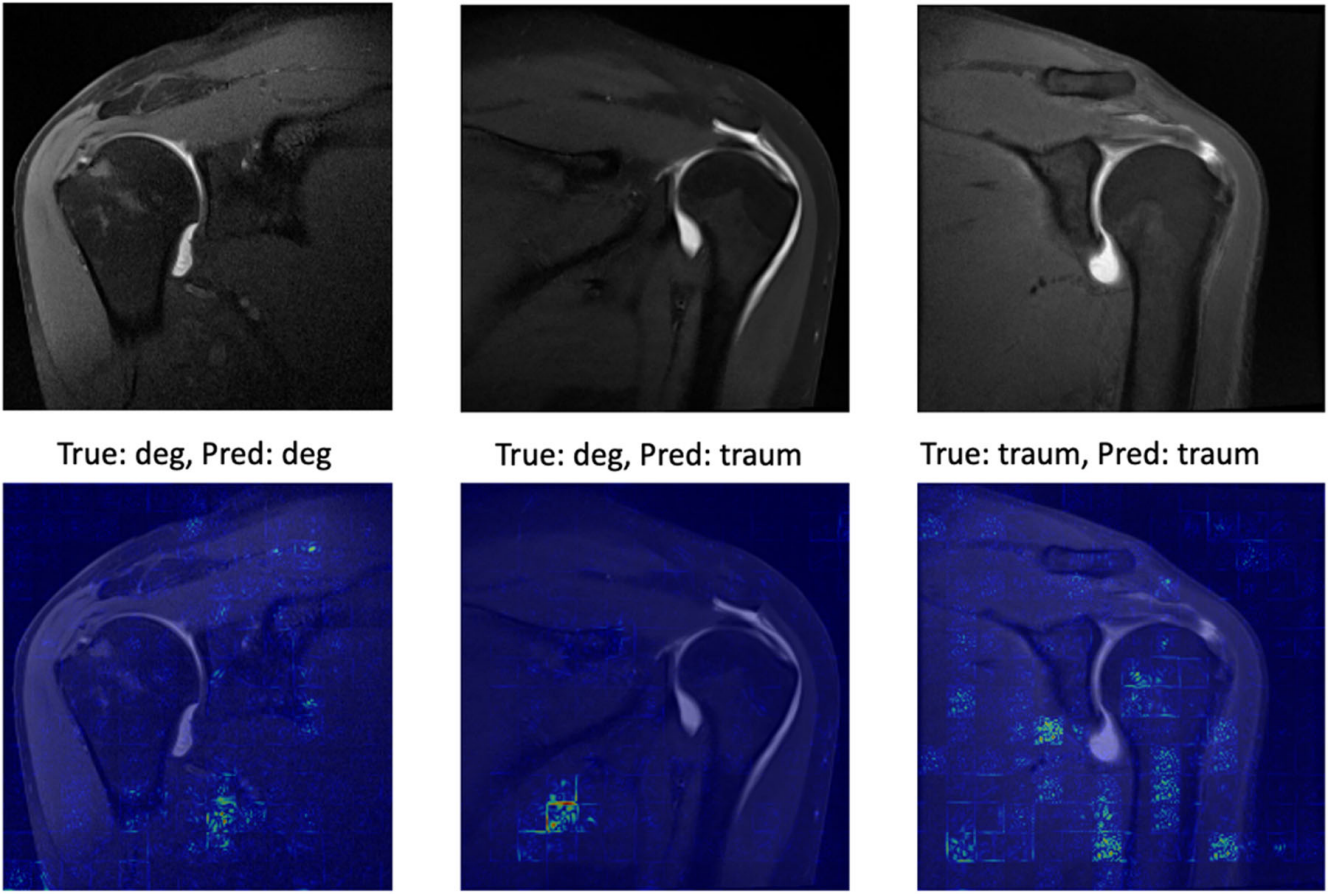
Saliency maps of correctly and incorrectly classified magnetic resonance imagings. There were no representative images of traumatic rotator cuff tears classified as degenerative.
